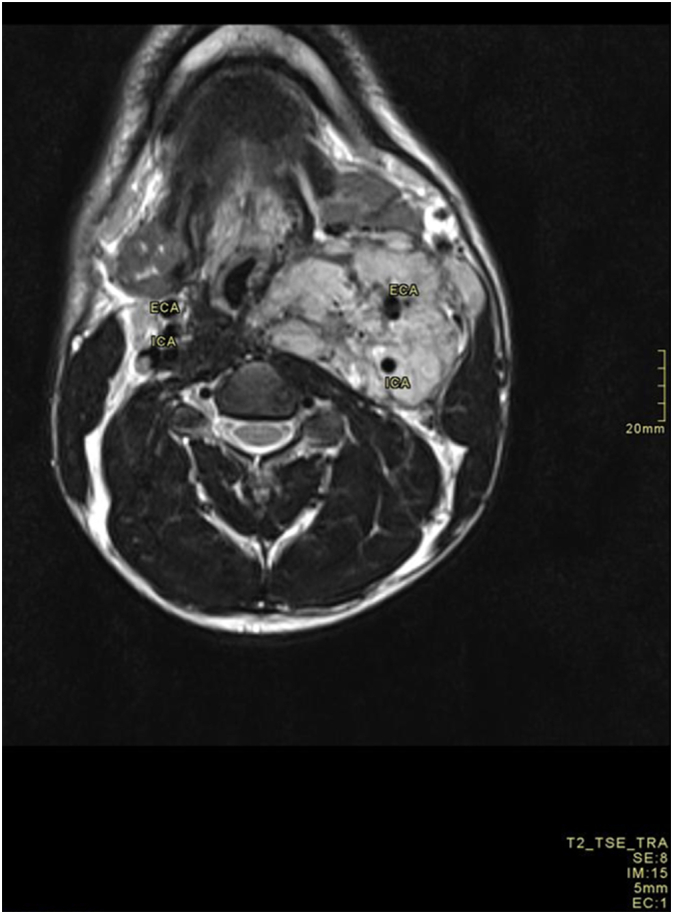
Cervical MRI images, T1 FS with contrast Axial demonstrating the vessel encasing left carotid paraganglioma.
